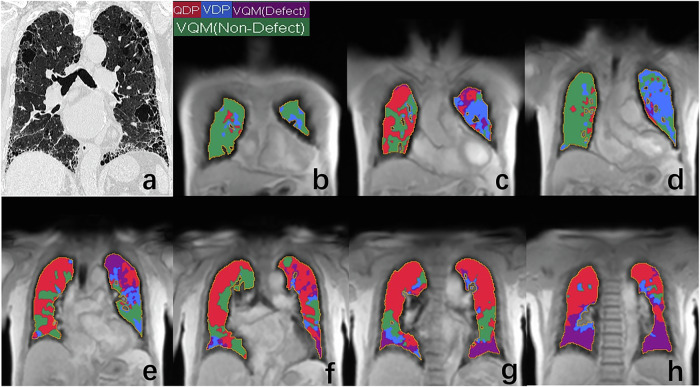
HRCT and PREFUL MRI ventilation/perfusion maps of a 67-year-old male patient with combined pulmonary fibrosis and emphysema. a The coronal HRCT indicating both honeycombing and predominantly in the lower zones and emphysema predominantly in the upper zones, b–h the coronal PREFUL MRI ventilation/perfusion maps of the whole lung. In ventilation/perfusion maps, red areas indicates QDPexclusive (percentage of areas with perfusion defects but without ventilation defects = 39.1%), blue areas represents VDPexclusive (percentage of areas with ventilation defects but without perfusion defects = 15.2%), green areas corresponds to VQMnon-defect (percentage of areas without perfusion defects or ventilation defects = 31.8%), and purple areas denotes VQMdefect (percentage of areas with concurrent perfusion and ventilation defects = 13.8%), prominent in the basal zones of bilateral lung. HRCT, high-resolution CT; PREFUL MRI, phase-resolved functional lung magnetic resonance imaging
